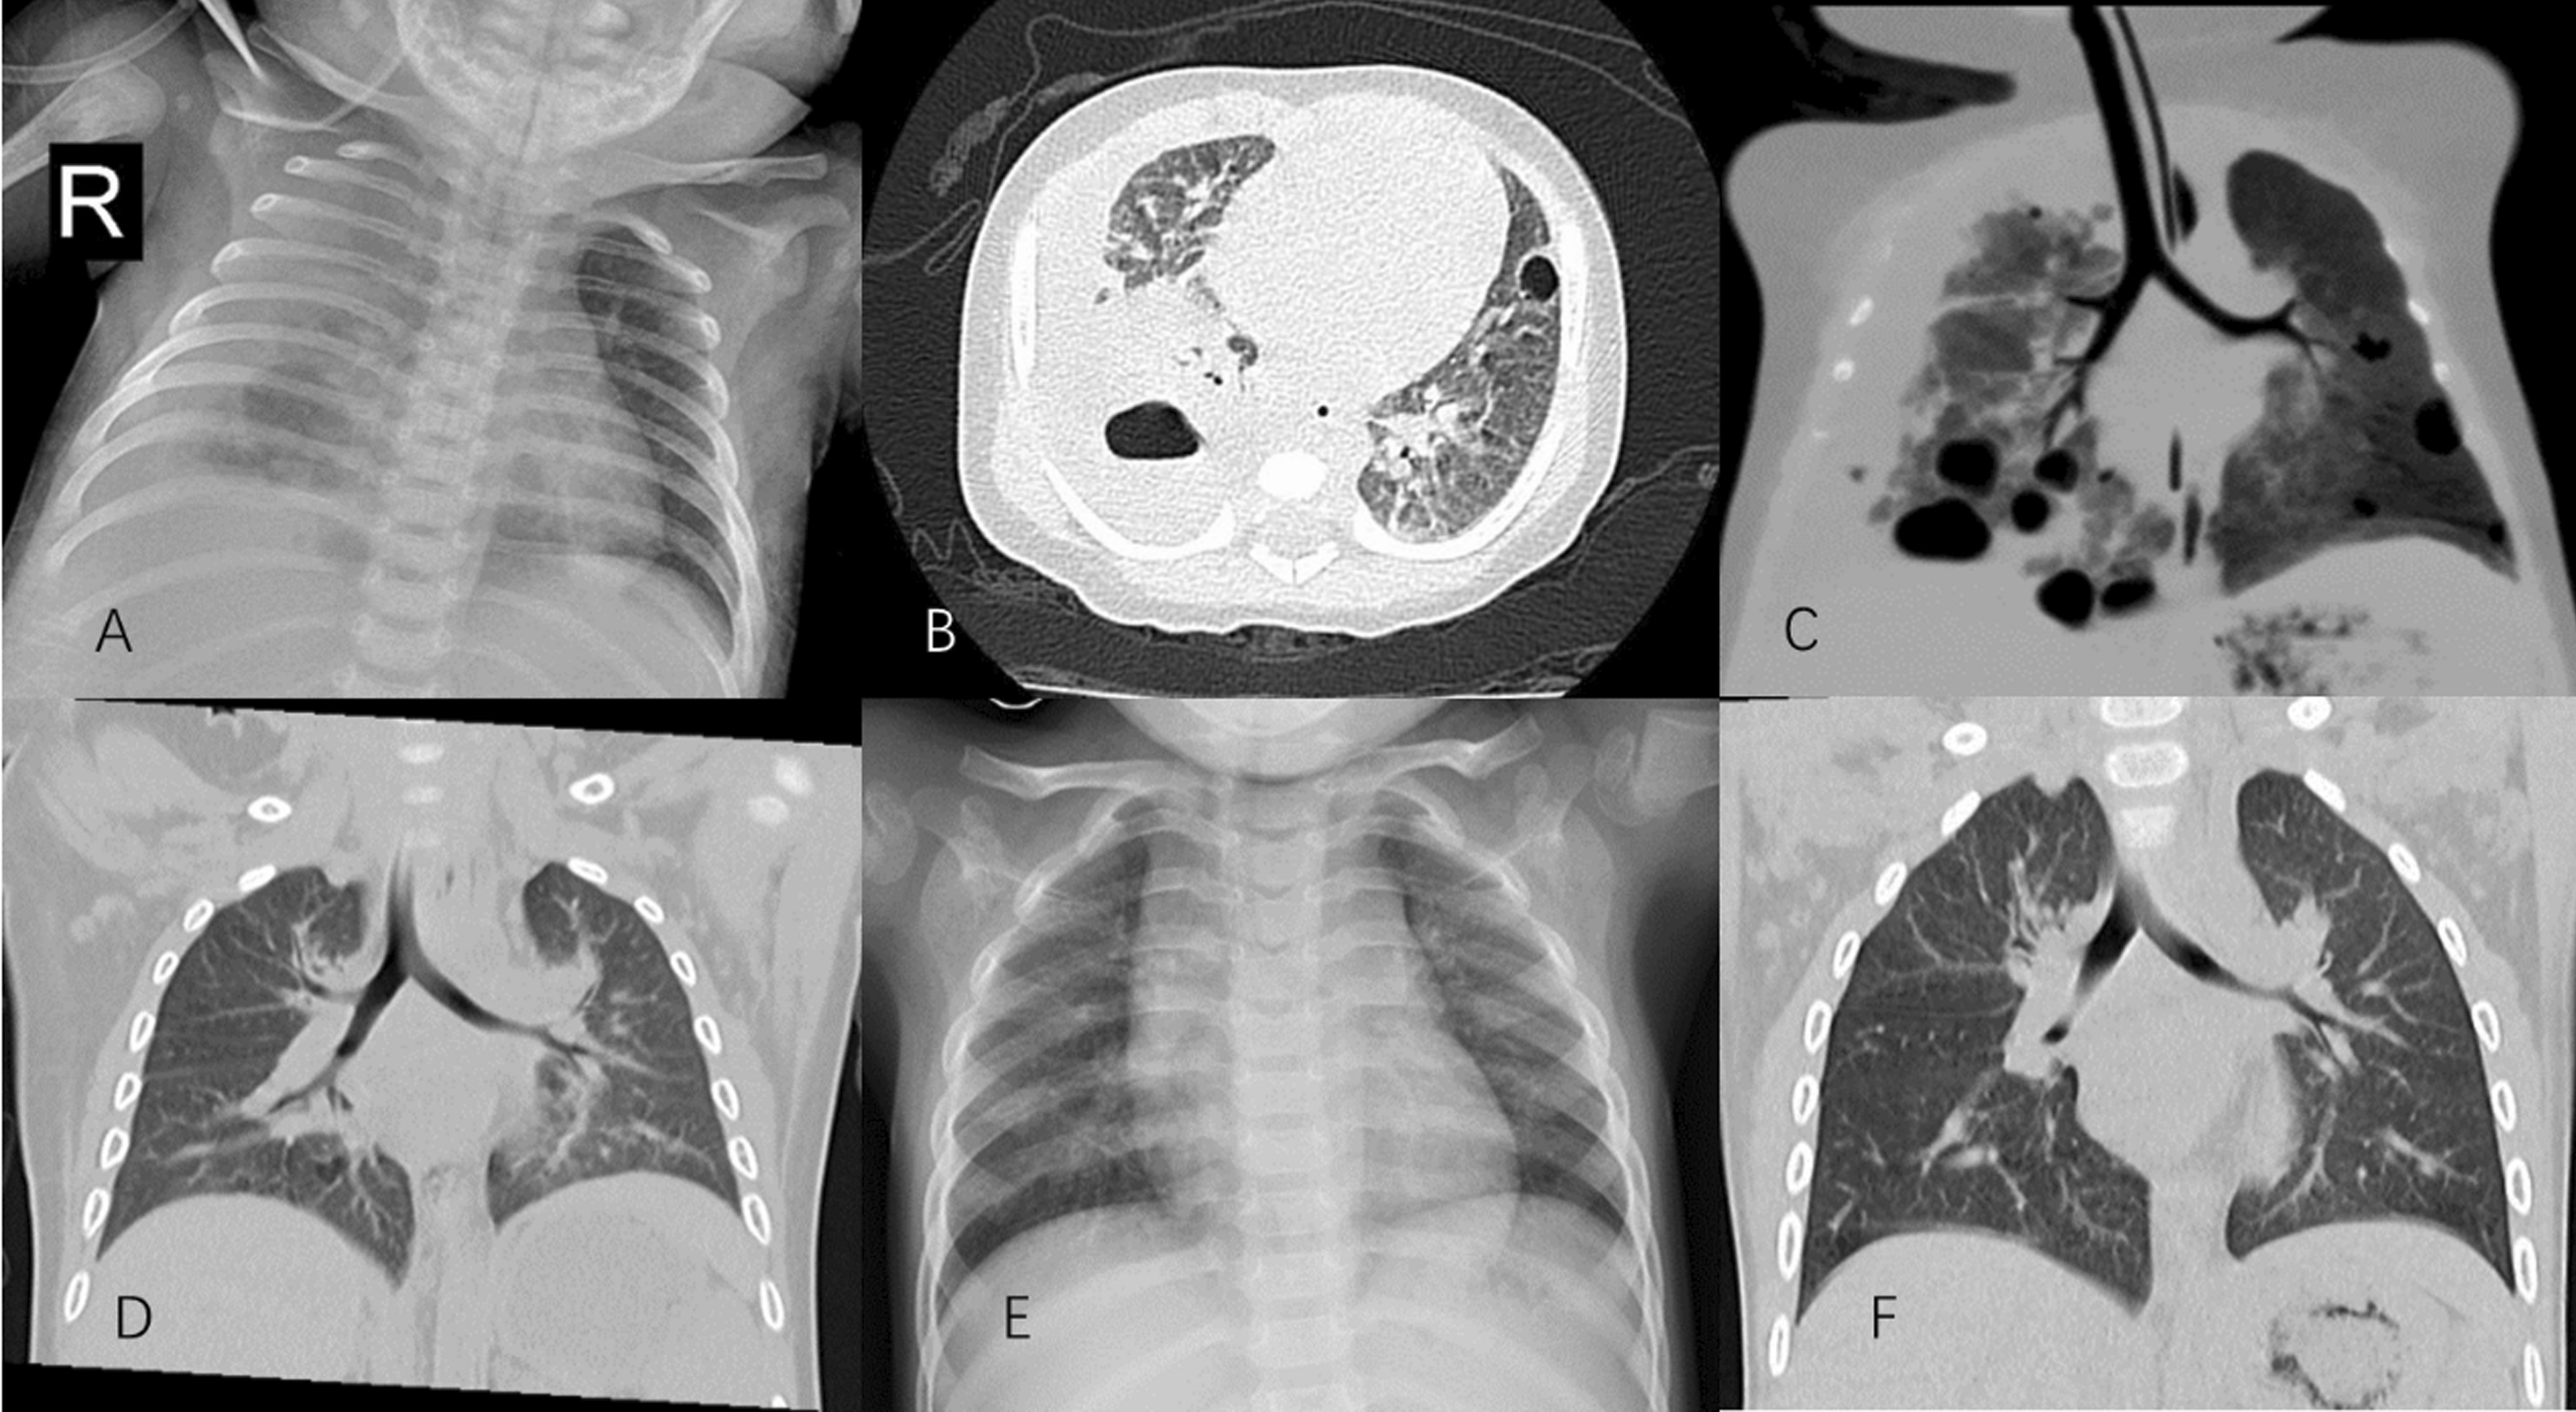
Changes on CXR and lung CT. A–C (Day7) CXR showing right pleural effusion and suspected lung abscess. CT revealing multiple pulmonary loculated abscesses, cavitation, consolidation, and right pleural effusions. D (CT 1 year later), E (CXR 2 years later) and F (CT three years later) showed significant improvement
